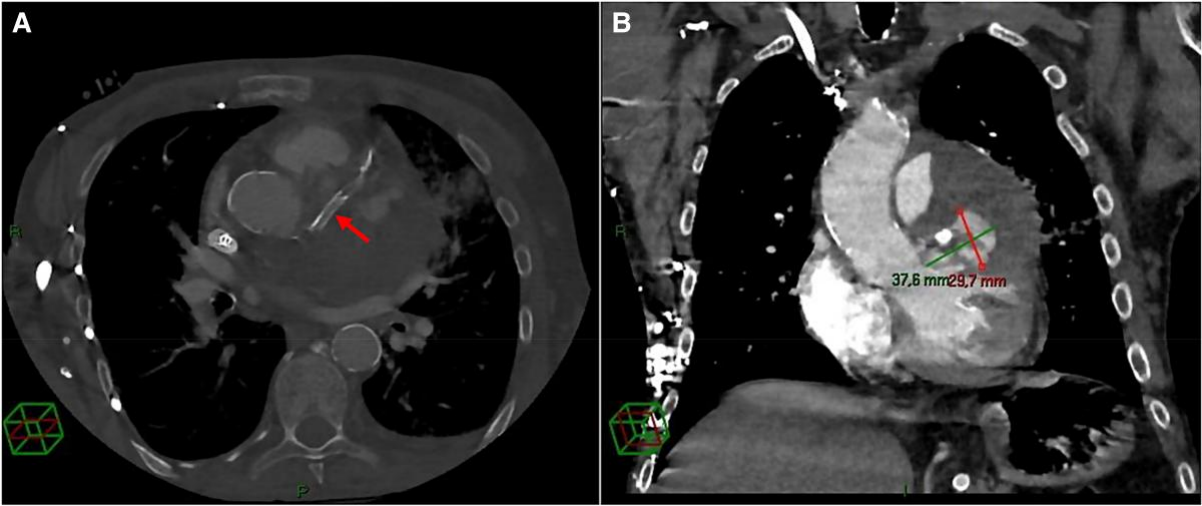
CT scan performed after re-admission for septic status. (*A*) Axial contrast-enhanced CT (bone window) demonstrating a predominantly thrombosed PSA arising from the proximal segment of the stented LAD. The covered stent (arrow) seems well expanded. (*B*) Coronal contrast-enhanced CT (mediastinal window) confirming the 37.8 × 29.7 mm PSA, which contains a substantial mural thrombus surrounding a contrast-filled core, supporting the existence of an active leakage into the PSA.
